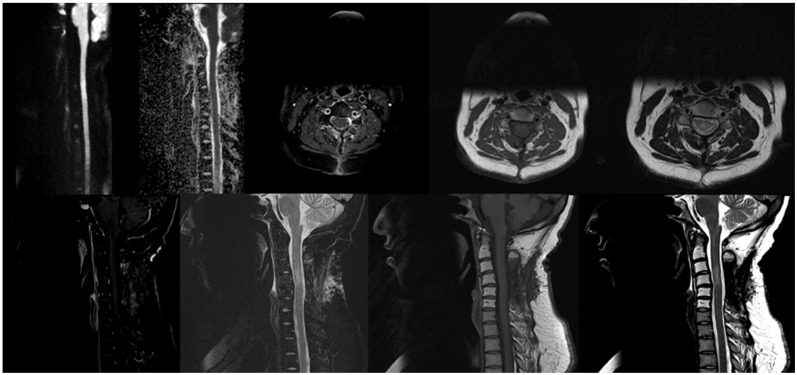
Follow up MRI Showed that there has been interval laminectomy performed at C3–C5 levels, resolution of the large intraspinal anterior epidural mass on the ventrolateral aspect of the canal at the level of C3–C4, improvement of the signal alteration within spinal cord due to cord myelopathy, no significant diffusion restriction, stable faint mild enhancement of C4 vertebra and new alteration on T1 and T2-weighted images at C2 to C6 vertebrae related to radiotherapy.
